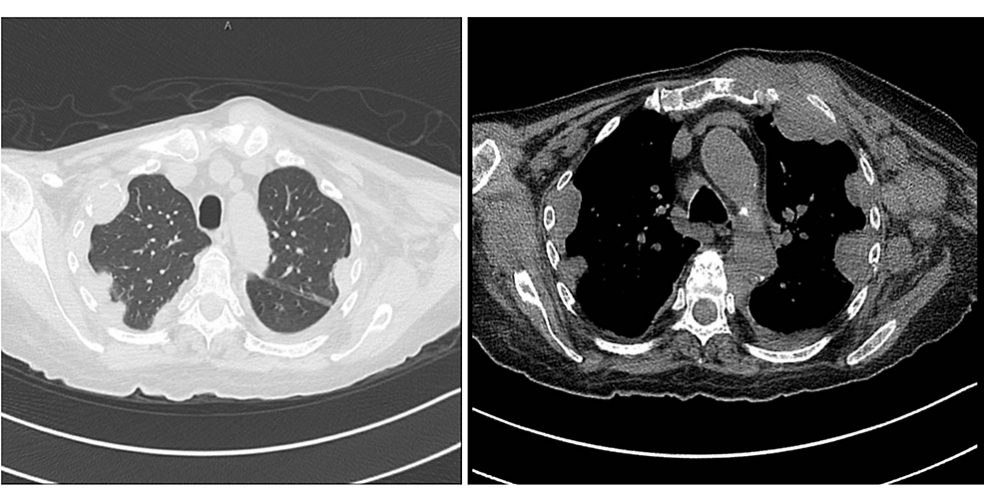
Chest CT scan showing numerous soft tissue masses along the bilateral thoracic osseous wall and subcutaneous tissues with interval development of multiple bilateral lung nodules, multiple lytic lesions throughout the thoracic wall (Patient 3)
